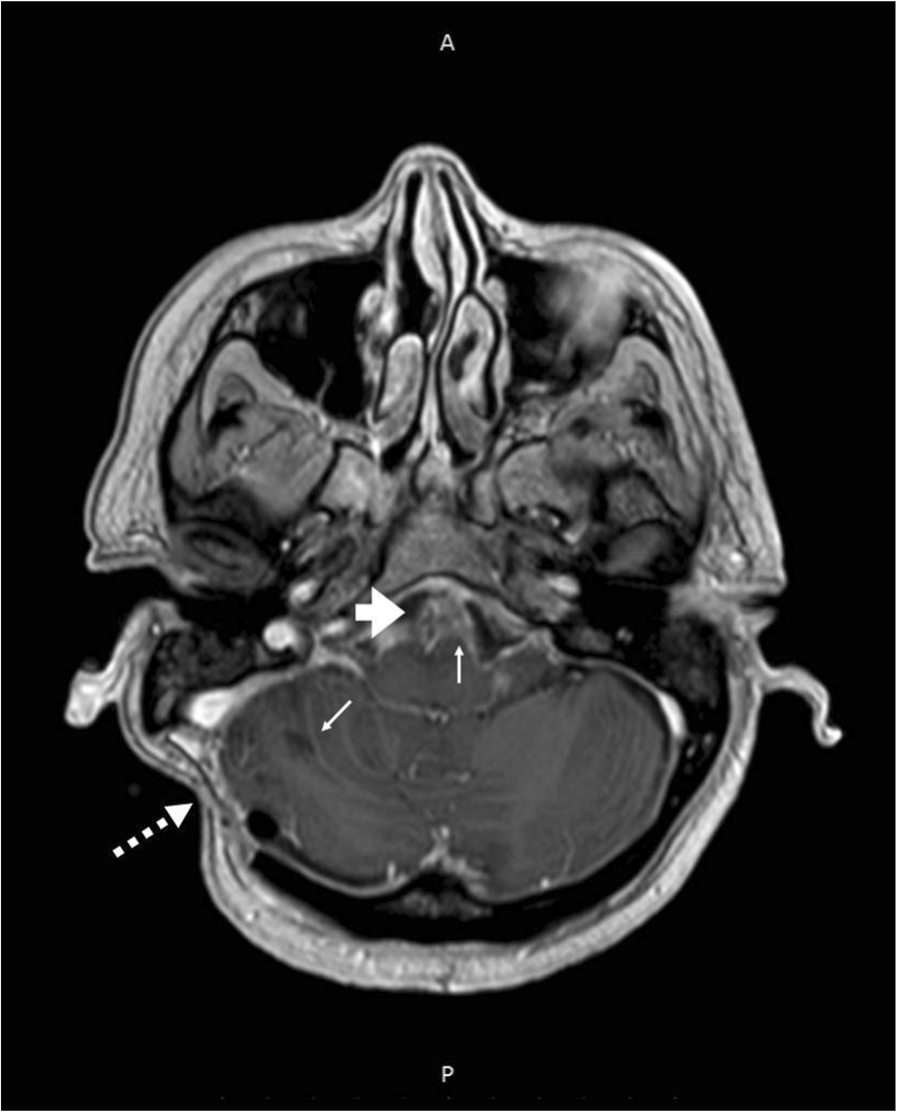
Axial Magnetic Resonance Imaging (MRI) at the posterior fossa level, showing extensive leptomeningeal enhancement near the anterior bulb contour and cerebellar folds (thin arrows). Heterogeneous material with enhancement near the pre-pontine cistern (thick arrow). Note the signs of surgical manipulation of the extra-cranial soft parts in the right occipital region (dashed arrow)
